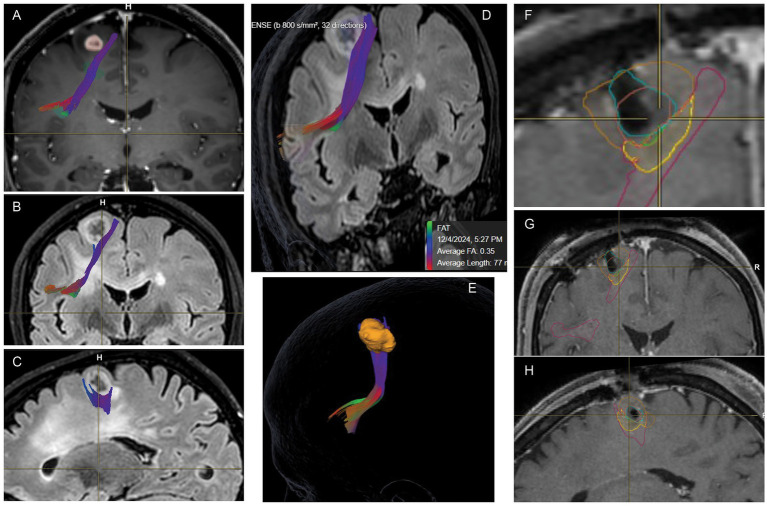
Preoperative and postoperative tractography studies. (A) Relationship between the tumor and FAT fibers; T1-weighted gadolinium-enhanced sequence, coronal view. (B, D) Relationship between the tumor and FAT fibers; FLAIR sequences, coronal view. (C) Relationship between the tumor and FAT fibers; FLAIR sequences, sagittal view. (E) Three-dimensional reconstruction showing the spatial relationship between the tumor (orange)and FAT, with the reconstructed bundle in purple/blue and the fiber endpoints color-coded in green and red according to fiber orientation. (F, G) Pre- versus post-operative comparison obtained by overlaying, on post-operative MR images the segmented volumes: tumor on pre-operative FLAIR sequence (orange), pre-operative FAT (magenta), portion of the FAT infiltrated by the tumor (yellow), post-operative resection cavity (cyan), and resected portion of the FAT (green); coronal view, with (F) magnified view and (G) overview. (H) Same overlay of pre- and post-operative volumes in sagittal view.
